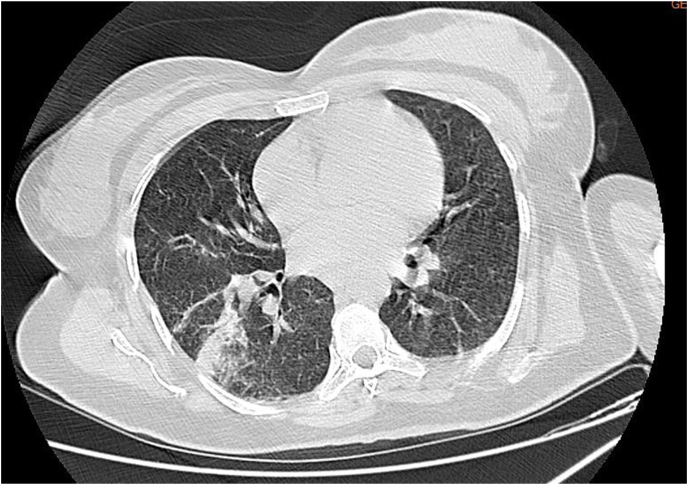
Axial lung window showing ground-glass opacities with interlobular septal thickening realizing a crazy paving pattern, as well as right lung peripheral pulmonary consolidation. SARS-Cov-2 RT-PCR positive, CT findings consistent with COVID-19 pneumonia CO-RADS 6.
